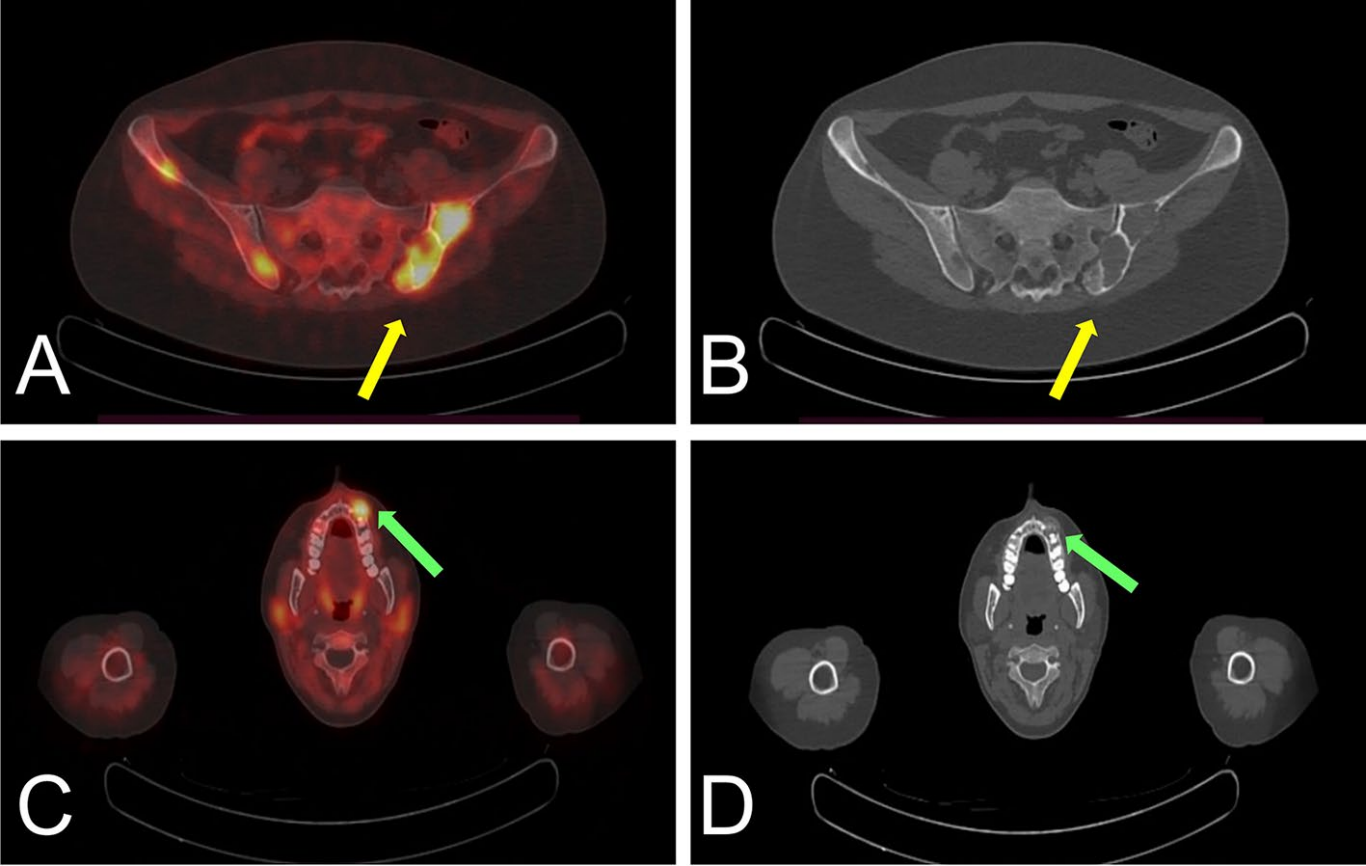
Axial [^18^F]fluorocholine PET/CT images (A) showing an avid lesion involving left iliac bone (SUVmax 7.9) and CT coregistration (B) (yellow arrows); axial [^18^F]fluorocholine PET/CT images (C) showing an avid lesion on left mandibular arch (SUVmax 5.6) and CT coregistration (D) (green arrows)
